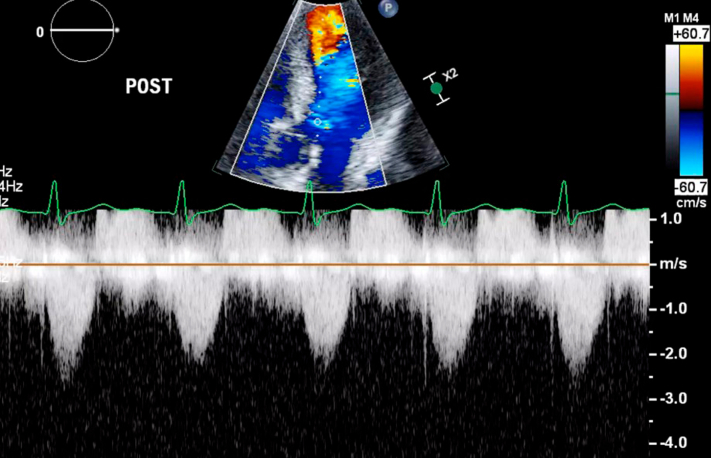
Athlete's Stress Left Ventricular Outflow Tract Gradient on 10 mg Mavacamten Continuous wave Doppler across the aortic valve displaying no significant left ventricular outflow tract gradient (<20 mm Hg).
